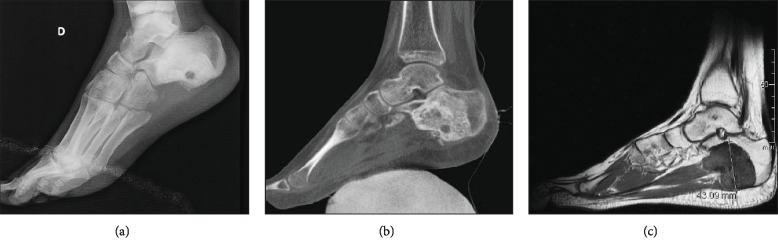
Osteosarcoma of the right calcaneus. (a) Lateral radiographs of the ankle. (b) CT scan of the right foot (sagittal). (c) MRI T1 FSE sagittal.
